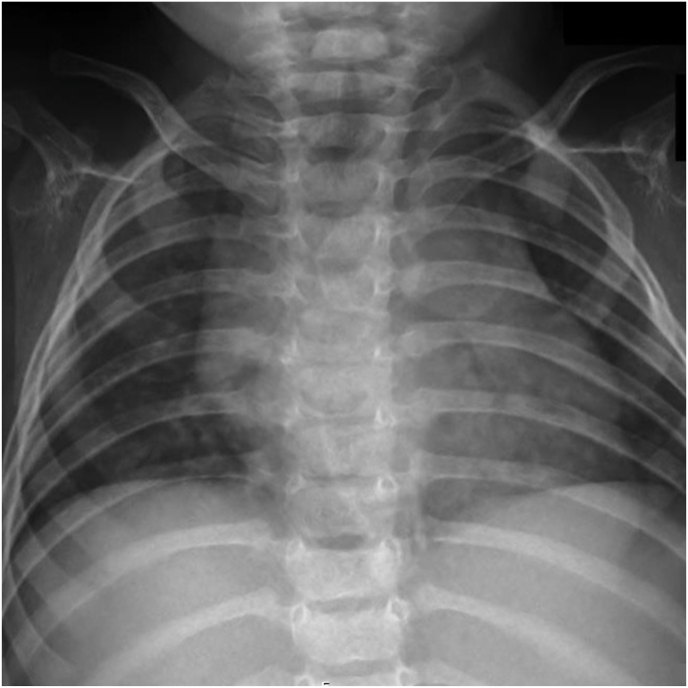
Chest X-ray of our patient showing ground glass appearances.
